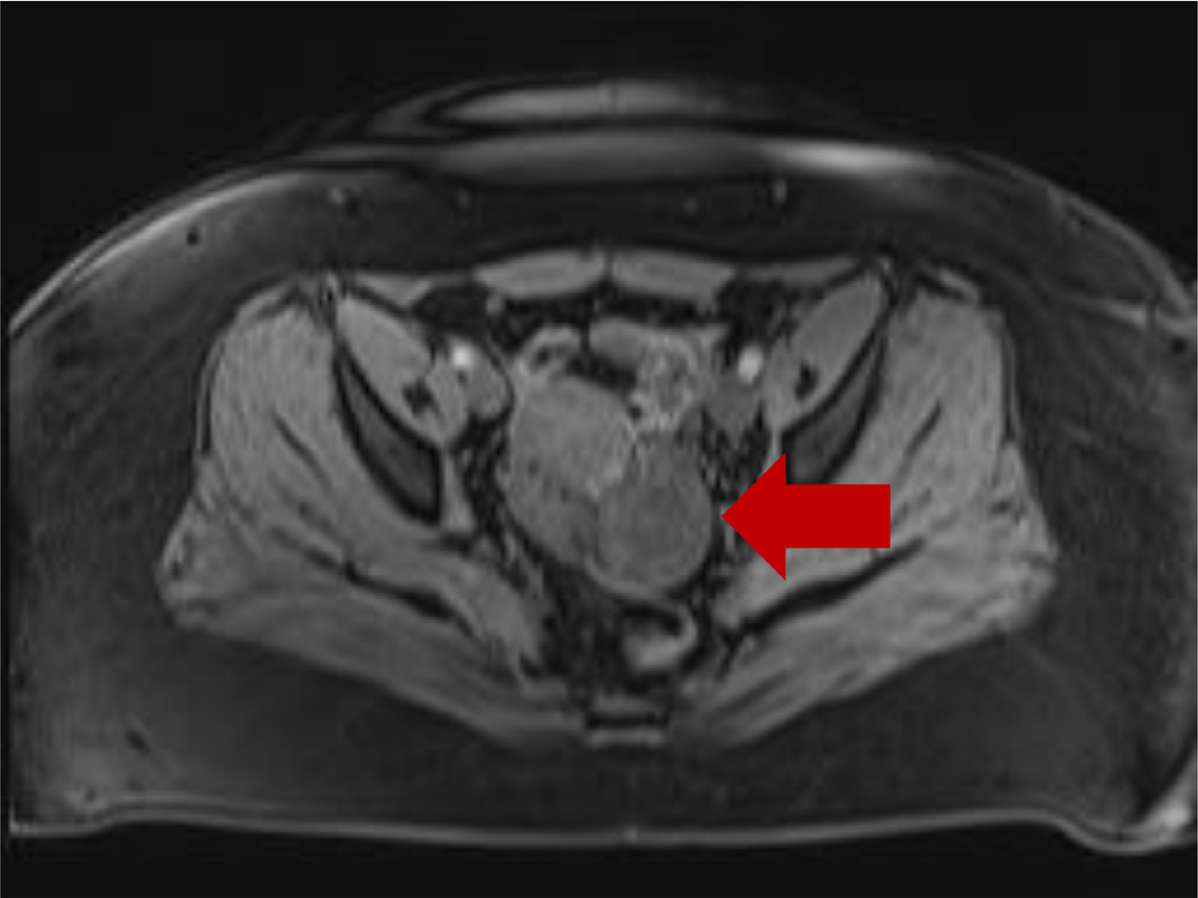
MRI of the pelvis. Axial T1-weigthed image demonstrates a minimally hypointense left ovarian mass.
